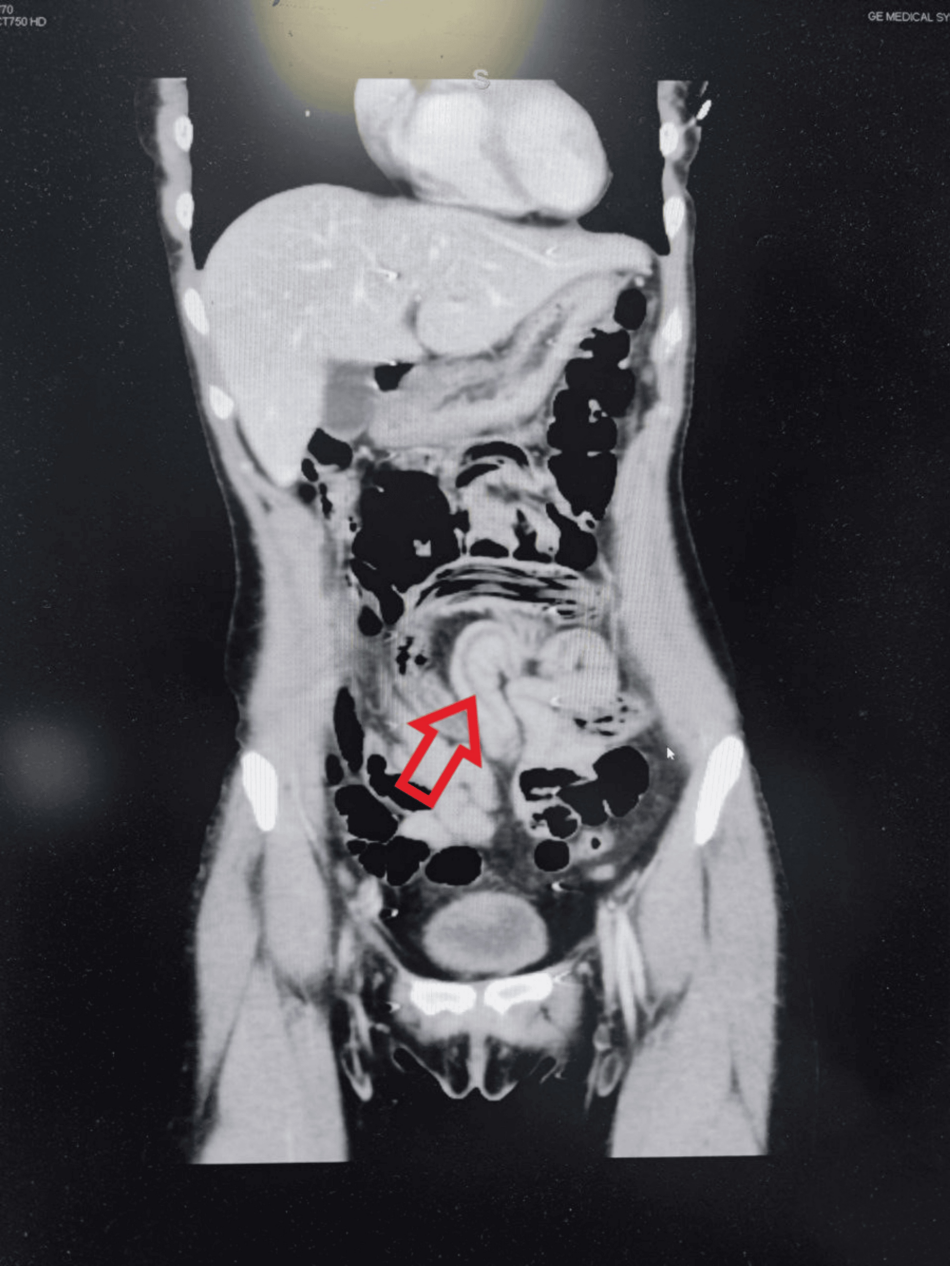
Abdominal computed tomography with intravenous contrast imaging showing air filled linear cavity changes seen in the small and large bowel loops.
